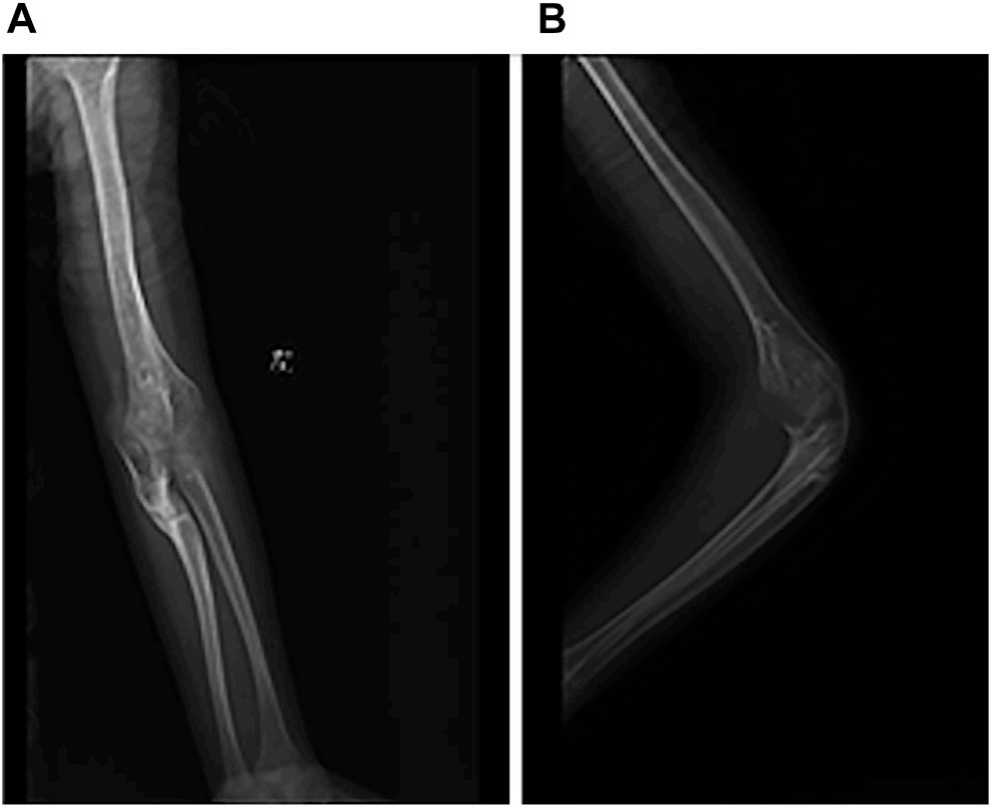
Radiograph of left elbow joint from conservative treatment. (A) AP view of elbow joint. (B) Latereal view of elbow joint.
